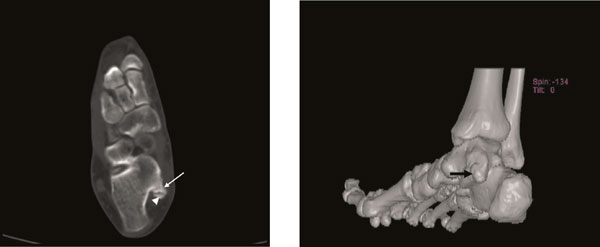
Axial CT scan (A) and three-dimensional (3D) imaging (B) taken at the 5-month postoperative of the foot shows increase in the lesion sizes (13 × 8 mm) (arrow: osteochondroma, arrowhead: cartilage cap).
